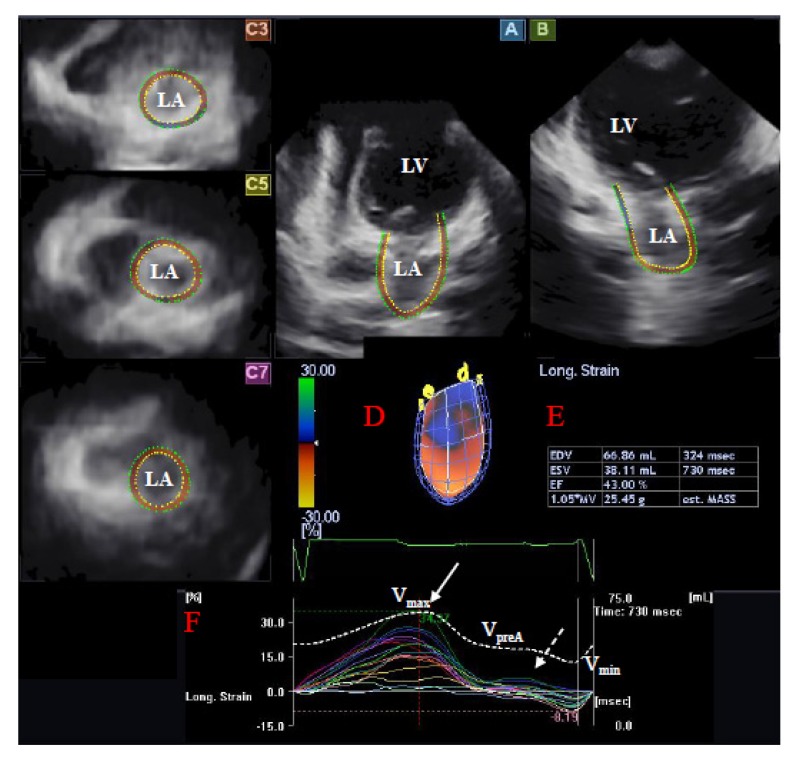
Three-dimensional (3D) speckle-tracking echocardiographic left atrial (LA) analysis using a full-volume dataset in an operated patient with dextro-transposition of the great arteries is presented: (A) apical four-chamber view, (B) apical two-chamber view, (C3) short-axis view at basal, (C5) mid- and (C7) superior left atrial level. The 3D model of the LA (D) and LA volumetric data (E) are also presented. Colored curves represent time–LA segmental strains, while dashed curve represents LA volume changes during the cardiac cycle with maximum (V_max_), minimum (V_min_) LA volumes, and LA volume before atrial contraction (V_preA_) (F). White arrow represents peak LA strain, while dashed arrow represents LA strain at atrial contraction (F). LA = left atrium, LV = left ventricle, V_max_ = maximum LA volume, V_min_ = minimum LA volume, V_preA_ = LA volume before atrial contraction.
